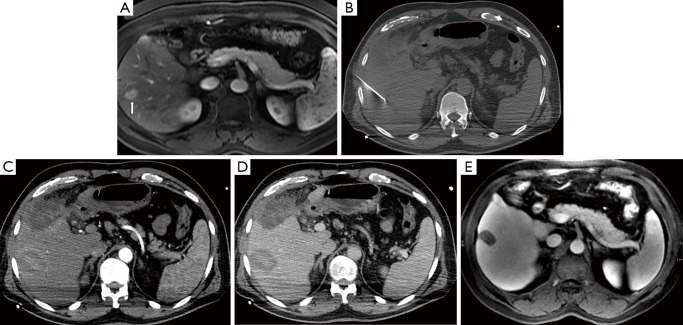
A 56-year-old male with hepatitis C complicated by hepatocellular carcinoma, not a surgical candidate, presenting with an isolated tumor in segment 6 of the liver. (A) T2 weighted axial MRI image demonstrates lesion in segment 6 (arrow). (B) Non-contrast axial CT image during procedure with applicator in the hypoattenuating mass. Immediate contrast-enhanced post-RFA axial CT images in arterial (C) and portal venous (D) phases show complete ablation in the area of the tumor. (E) T2 weighted axial MRI image at two year follow-up demonstrates complete necrosis of the segment 6 tumor. RFA, radiofrequency ablation.
